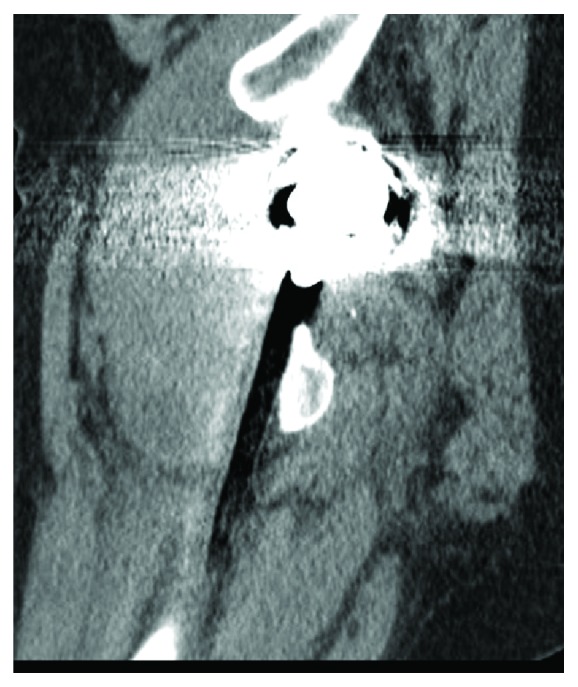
Sagittal section of CT scan showing the hematoma.
